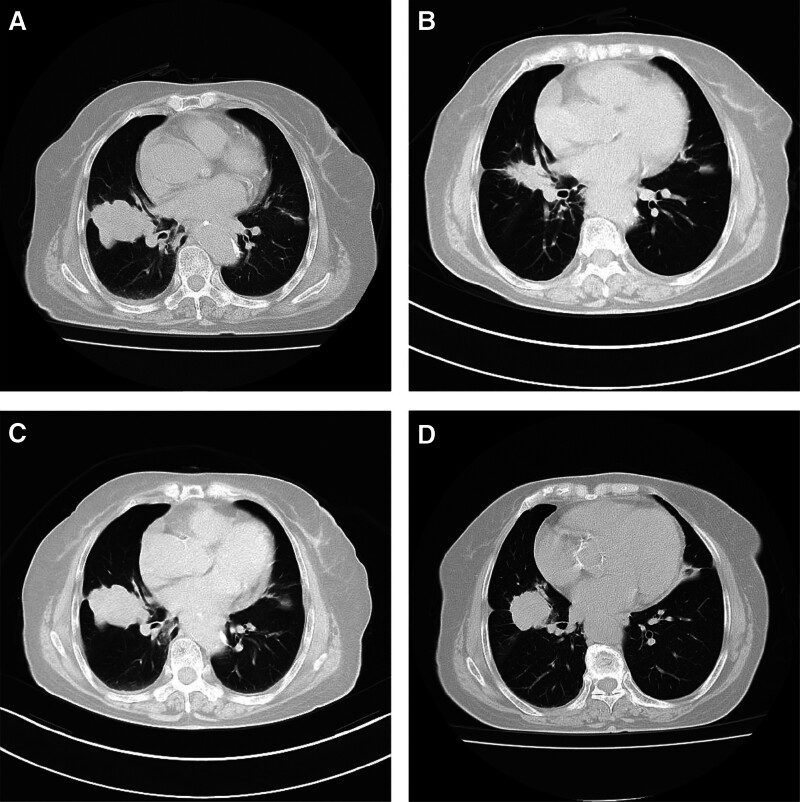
The CT imaging indicates that the boundaries of the right lung mass are indistinct, with adjacent pleural thickening and adhesion. (A) November 22, 2023: The largest cross-sectional area of the right lung mass measured approximately 59 mm × 43 mm (slice 29/3). The mass displayed a lobulated contour with heterogeneous density; no calcifications or cavitations were noted. There was invasion into the adjacent pleura, and the lesion showed marked heterogeneous enhancement on contrast-enhanced scans. (B) January 10, 2024: A follow-up CT scan of the right middle lobe showed a significant reduction in the size of the irregular mass, now measuring approximately 32 mm × 27 mm. (C) February 2024: Subsequent imaging revealed an increase in the size of the irregular mass in the right middle lobe, now measuring approximately 47 mm × 36 mm. The mass retained a lobulated appearance with heterogeneous internal density, without evidence of calcification or cavitation, and continued invasion into the adjacent pleura. Contrast-enhanced scans demonstrated pronounced heterogeneous enhancement of the tumor. (D) April 7, 2024: Compared to the findings from February 2024, there was no significant enlargement of the right lung mass.
